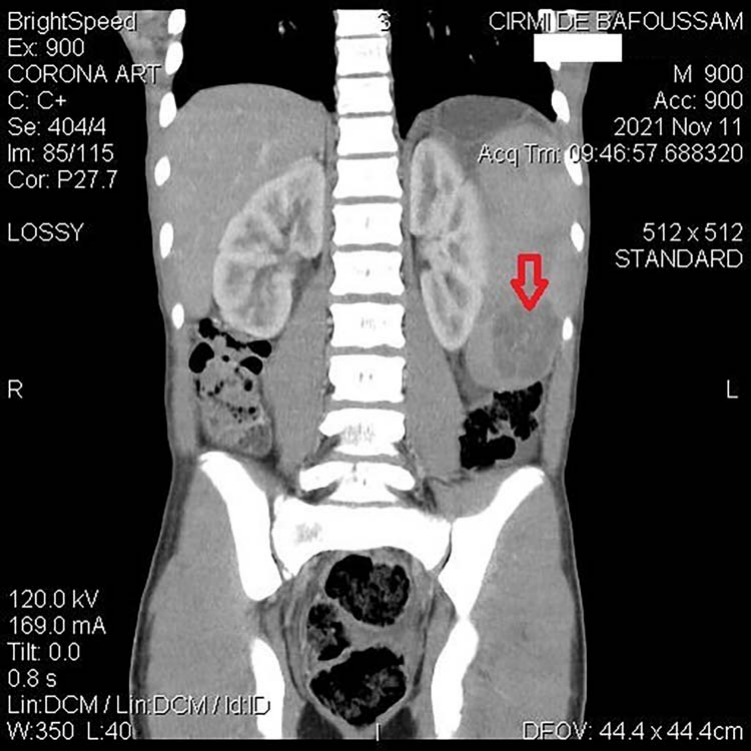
Abdominal CT demonstrating a contrast-enhanced hypodense mass in the spleen.
